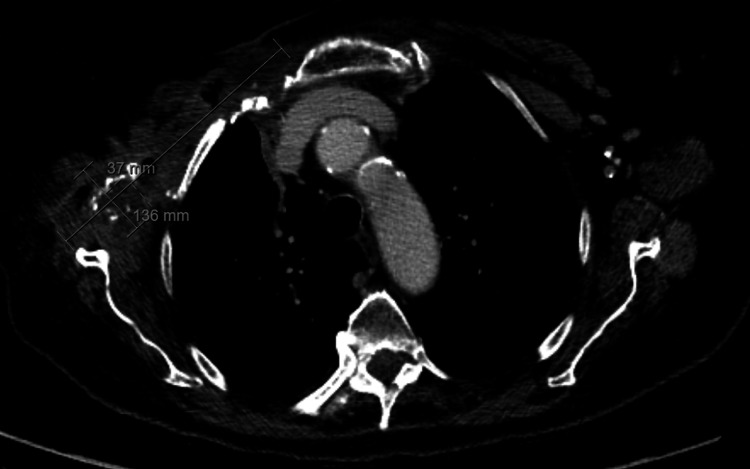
Axial computed tomography showing right anterior thoracic abscess and left sternoclavicular septic arthritis A right-sided fluid-and-gas collection measuring approximately 136 × 37 millimetres (anteroposterior × transverse) is seen in the upper anterior hemithorax, extending inferiorly toward the right axillary region, compatible with an abscess. Axial computed tomography also demonstrates features suggestive of septic arthritis of the left sternoclavicular joint, including intra-articular fluid, internal gas foci and marked subcutaneous fat stranding.
